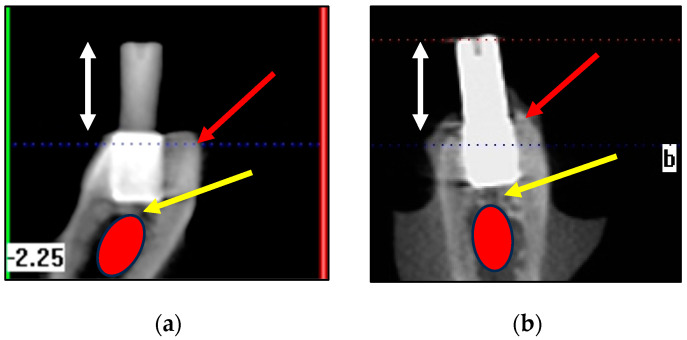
Examples of CBCT bucco-lingual views (a,b). CBCT confirmed good crestal bony coverage (red arrows). These bucco-lingual views highlighted the proximity (yellow arrows) of the “apical” base and the superior aspect of the inferior alveolar nerve canal (red ellipses). Given that the length of this implant is 5.25 mm, it emerged that a longer implant would be anatomically precluded. White arrows represent relative 6 mm lengths. Buccal orientation in these cross sections: “b”.
